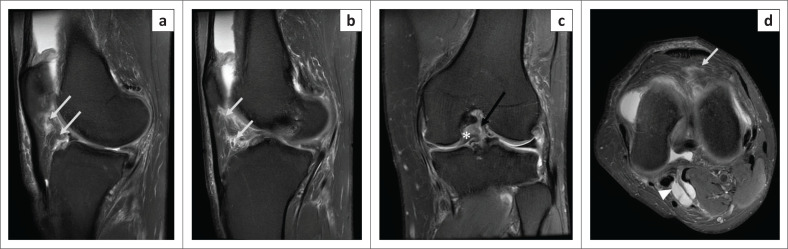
Magnetic resonance imaging of infrapatellar plica sprain in a 45-year-old male with a history of a fall: serial PDFS oblique sagittal images (a and b), oblique coronal PDFS (c) and axial PDFS (d) demonstrates hyperintense signal along the entire course of infrapatellar plica (white arrows in a, b and d) having ragged margins, with oedema in the surrounding Hoffa’s fat pad and oedema in the prepatellar subcutaneous plane (d). Note mild sprain in the middle third of the postero-lateral bundle of the anterior cruciate ligament in (c) (black arrow), associated bucket handle tear of the medial meniscus with the displaced fragment lying within the inter-condylar notch (c) (asterisk), small Baker’s cyst posteriorly (d) (arrow head).
